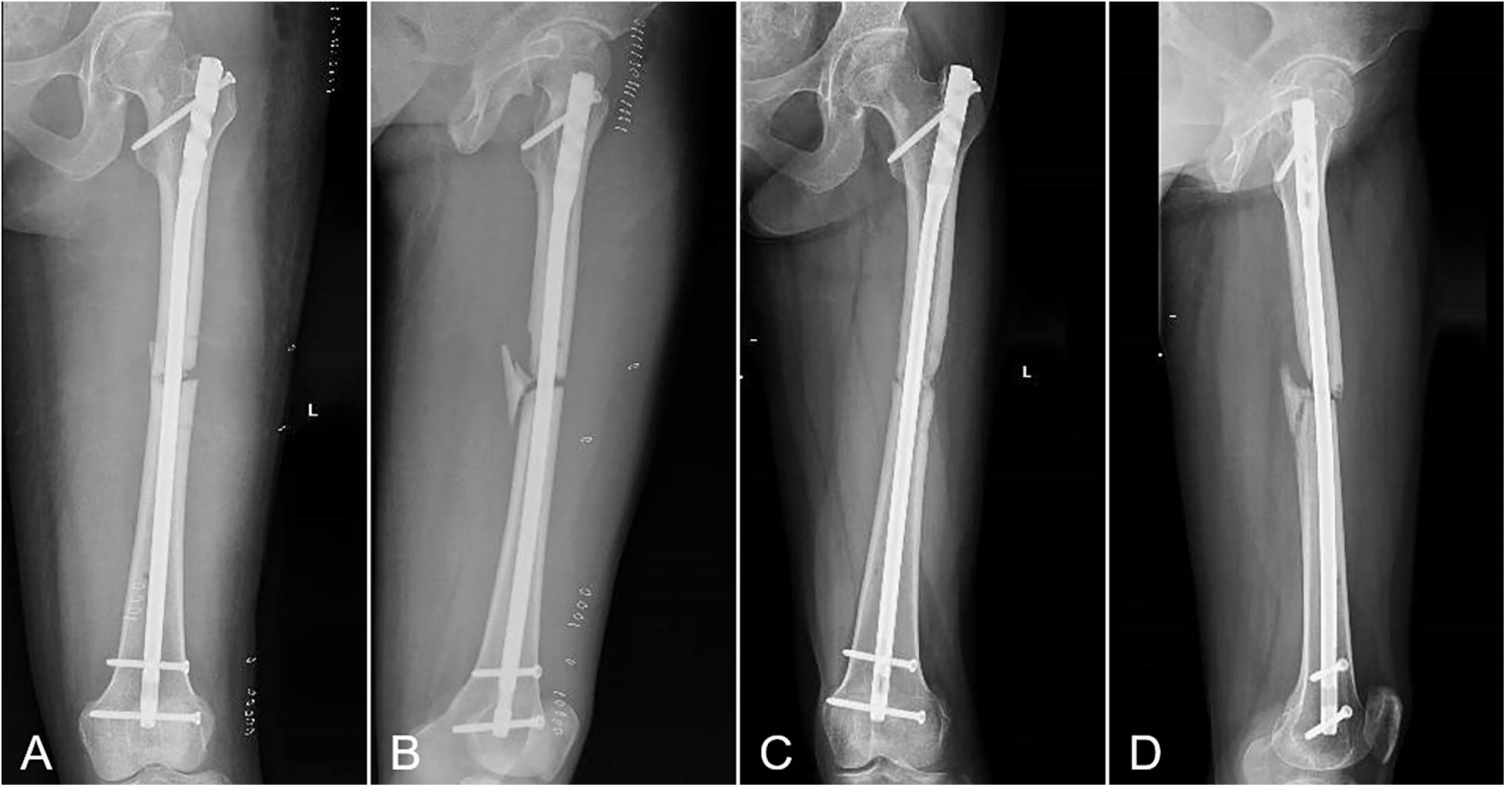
A case of fracture nonunion. (A,B) Immediate postoperative anteroposterior (A) and lateral (B) radiographs of a patient who subsequently developed nonunion. (C,D) Follow-up anteroposterior (C) and lateral (D) radiographs of the patient at 10 months postoperatively, confirming nonunion with no bridging callus across the fracture gap.
